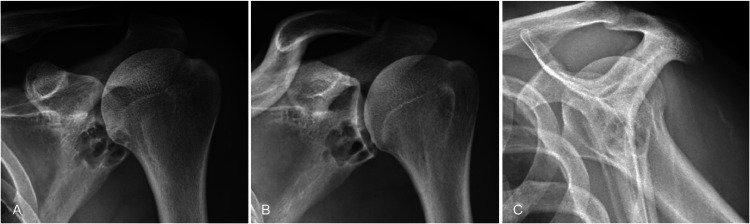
Left shoulder X-rays obtained in (A) anteroposterior, (B) Grashey, and (C) lateral views demonstrate the lucent lesion in the subchondral bone of the inferior glenoid.
